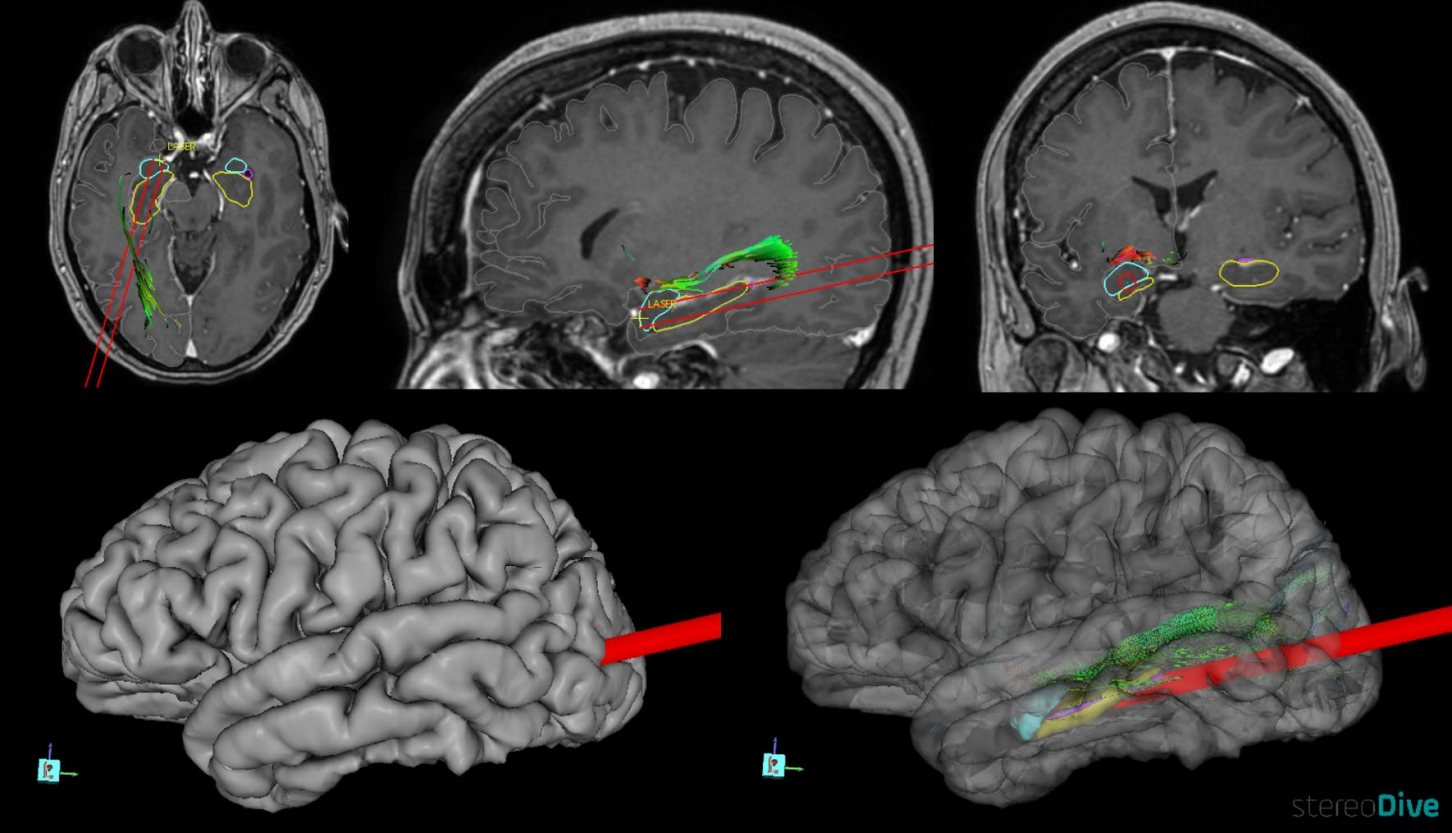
3D planning imaging in a HS case. Trajectory planning is performed using a Fig. 1. 3T MRI with double contrast and SD tractography, using StereoDiver® software
